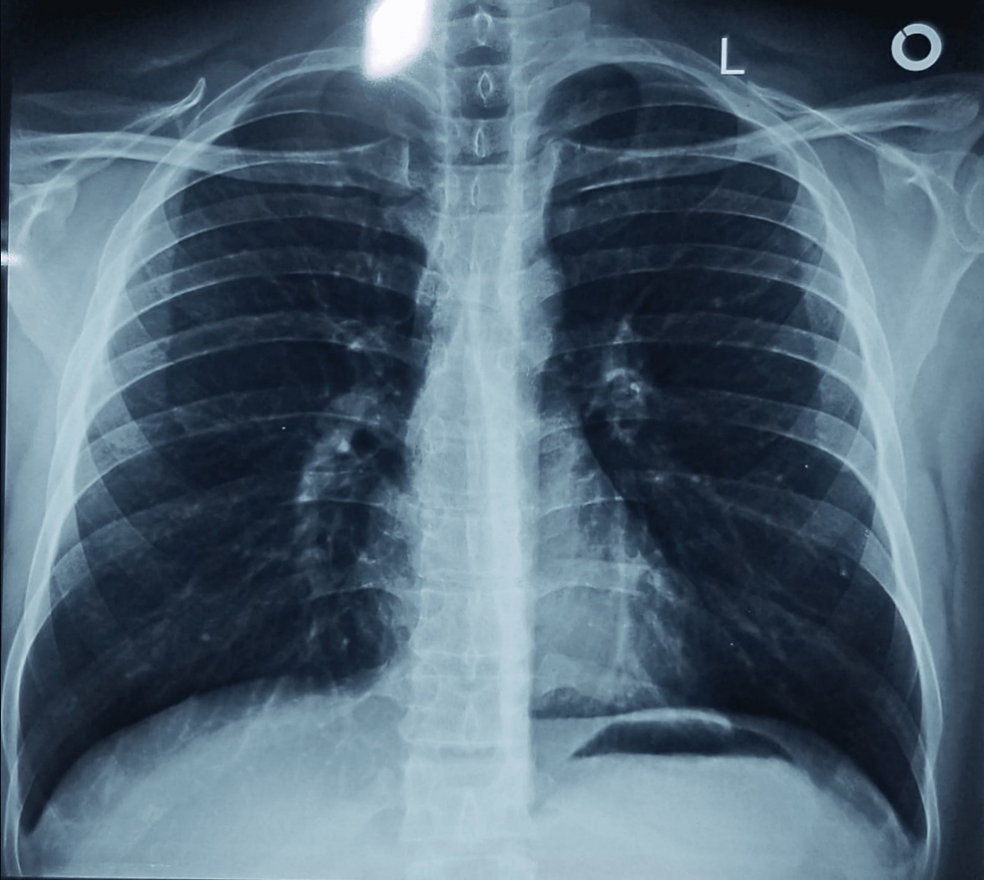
Chest X-ray showing bilateral pulmonary hyperinflation with perihilar infiltrates
